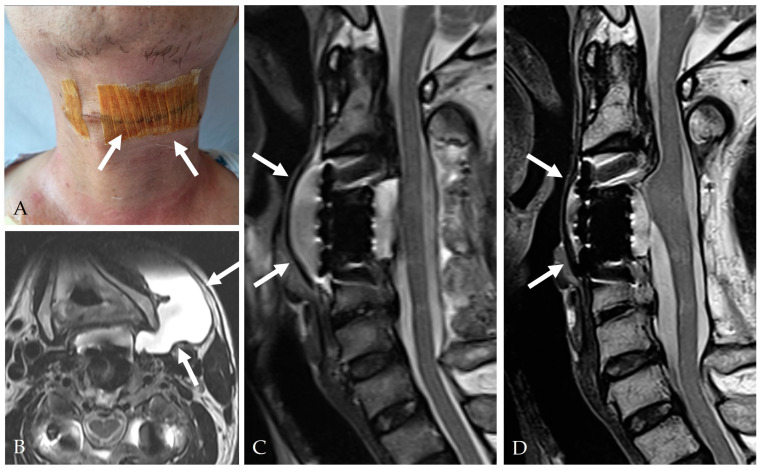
At postoperative 13 days, the patient complained of anterior left side neck swelling (A). Follow-up MRI showed an extensive CSF collection (B,C). Revision surgery was performed for CSF drainage and augmented closure of the CSF leakage site with Tacocom and sealant. In addition, lumbar drainage was performed for 5 days. One month after revision surgery, follow-up MRI showed healing of the dural tear and a small remnant of CSF (D).
